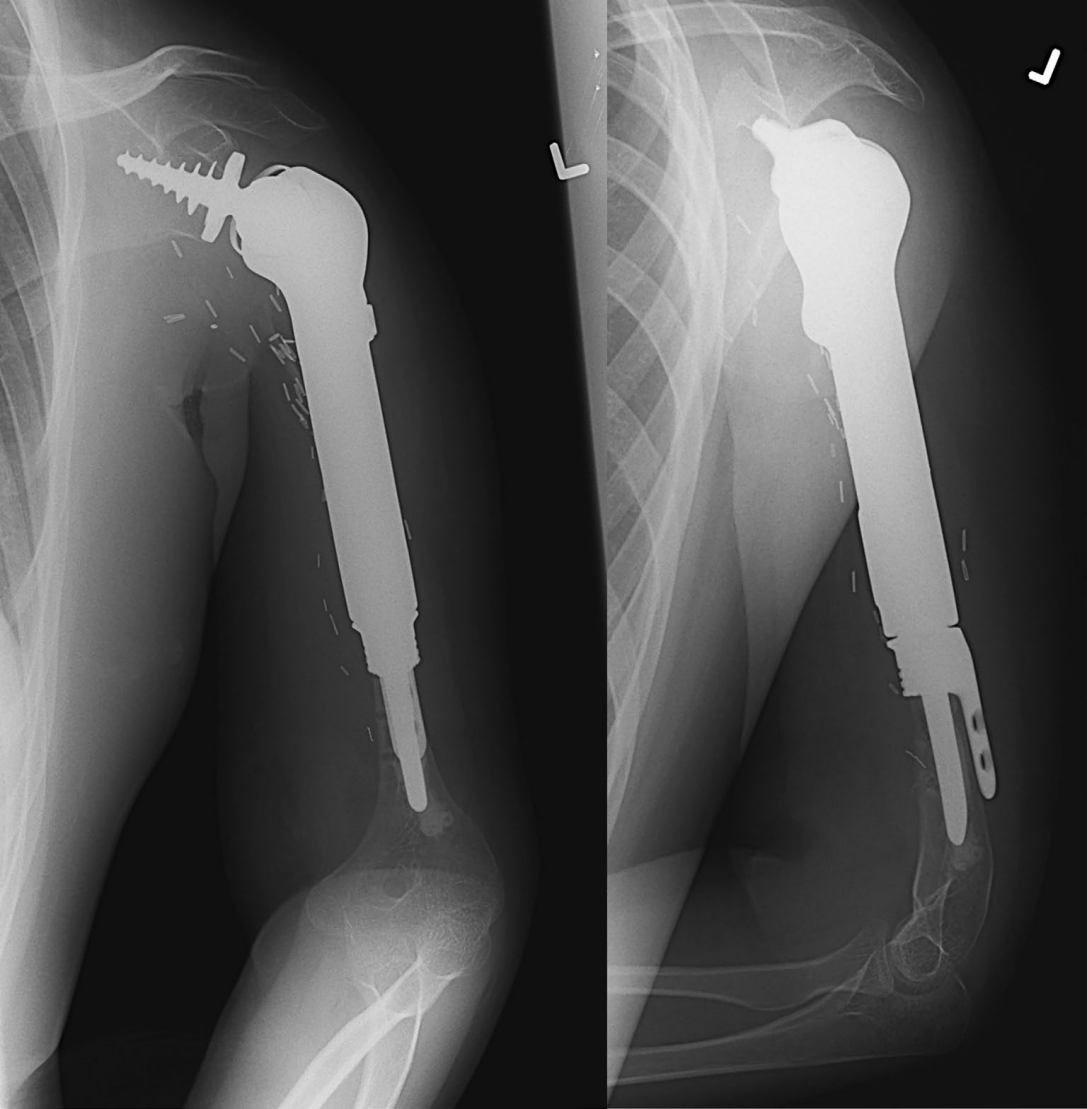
Immediate postoperative plain radiographs of patient in Figure 7 with a proximal humerus replacement with a growing (extendable) endoprosthesis and a HA‐coated stem collar with an extracortical plate.
